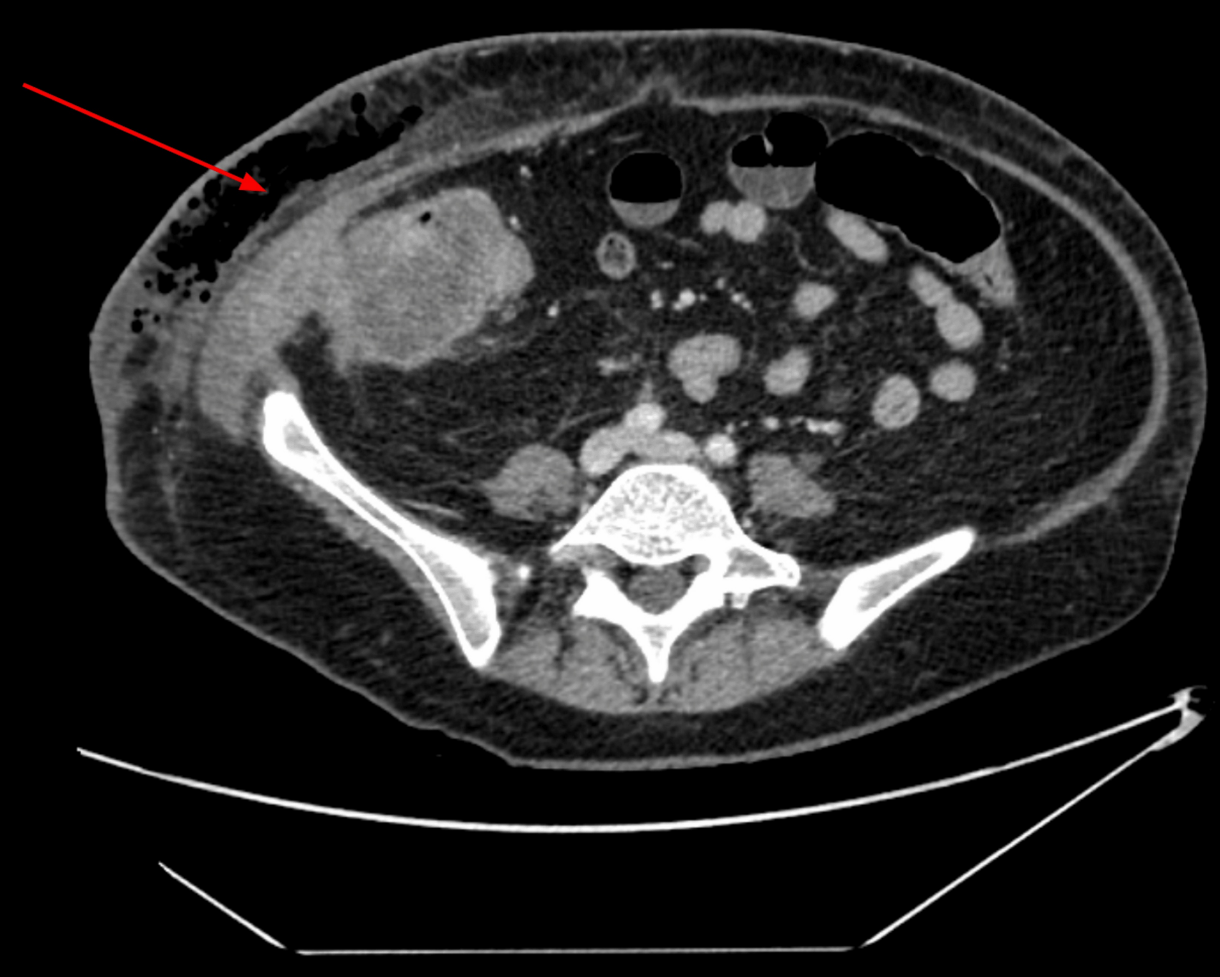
CT scan with IV contrast: axial image showing an inflamed colon with masslike thickening of the cecum concerning for primary colonic malignancy contiguous with the abdominal wall fluid collection and subcutaneous emphysema.
